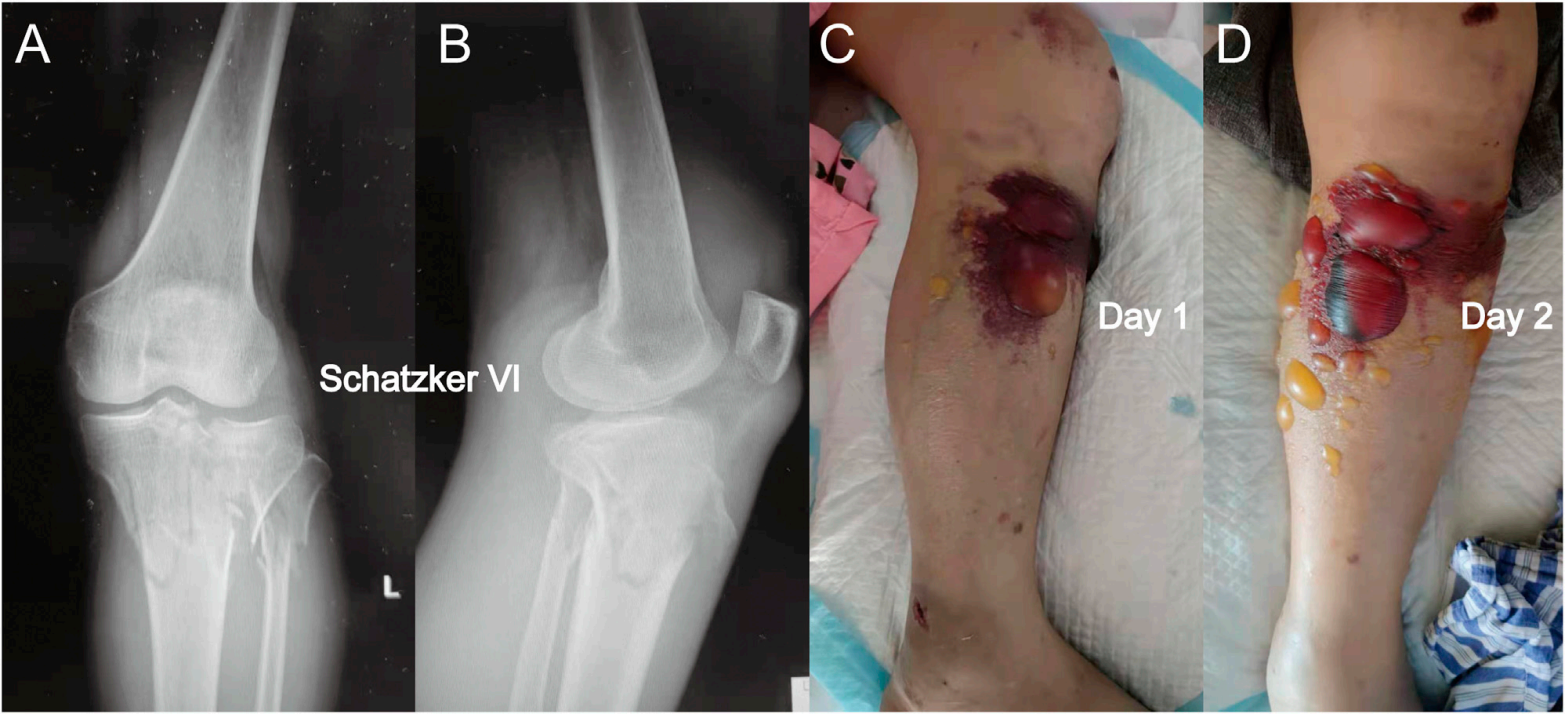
(A) Anterior-posterior X-rays of another Schatzker VI tibial plateau fracture. (B) Lateral view of this fracture. (C) Fracture appearance with a blood-filled blister. The blood-filled blister was larger than the serous blister observed in Figure 1. (D) Blister became larger after 1 day, and a similar phenomenon in which the intracompartmental pressure decreased after the appearance of the blood-filled blister was observed.
